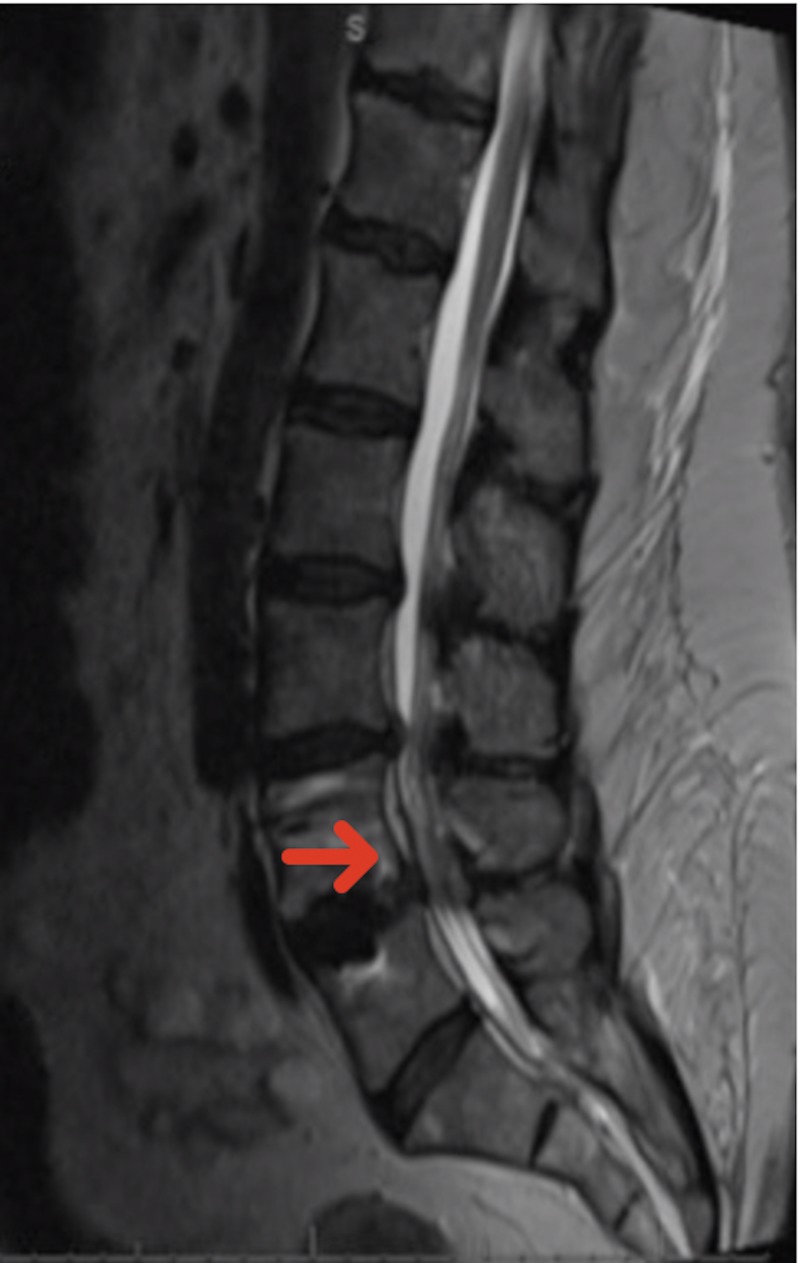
Post-operative sagittal lumbar MRI demonstrating improvement of the previous L4/5 spondylolisthesis and canal stenosis.
